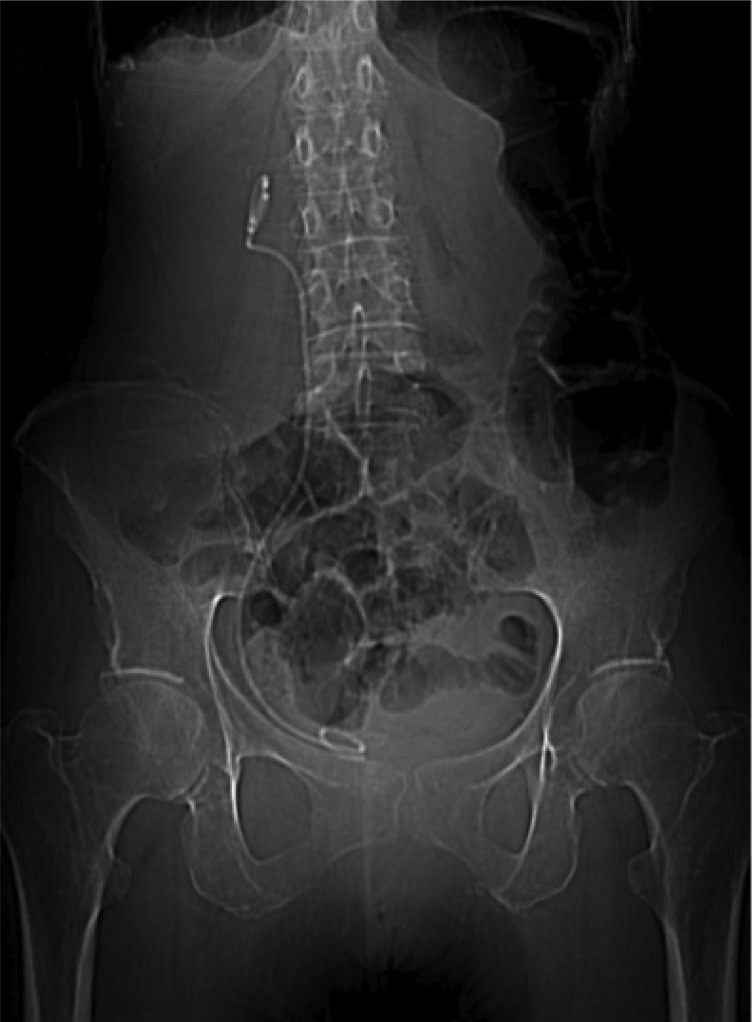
Plain abdominal radiograph demonstrating a right ureteral stent in proper position and a straightened ureter.
